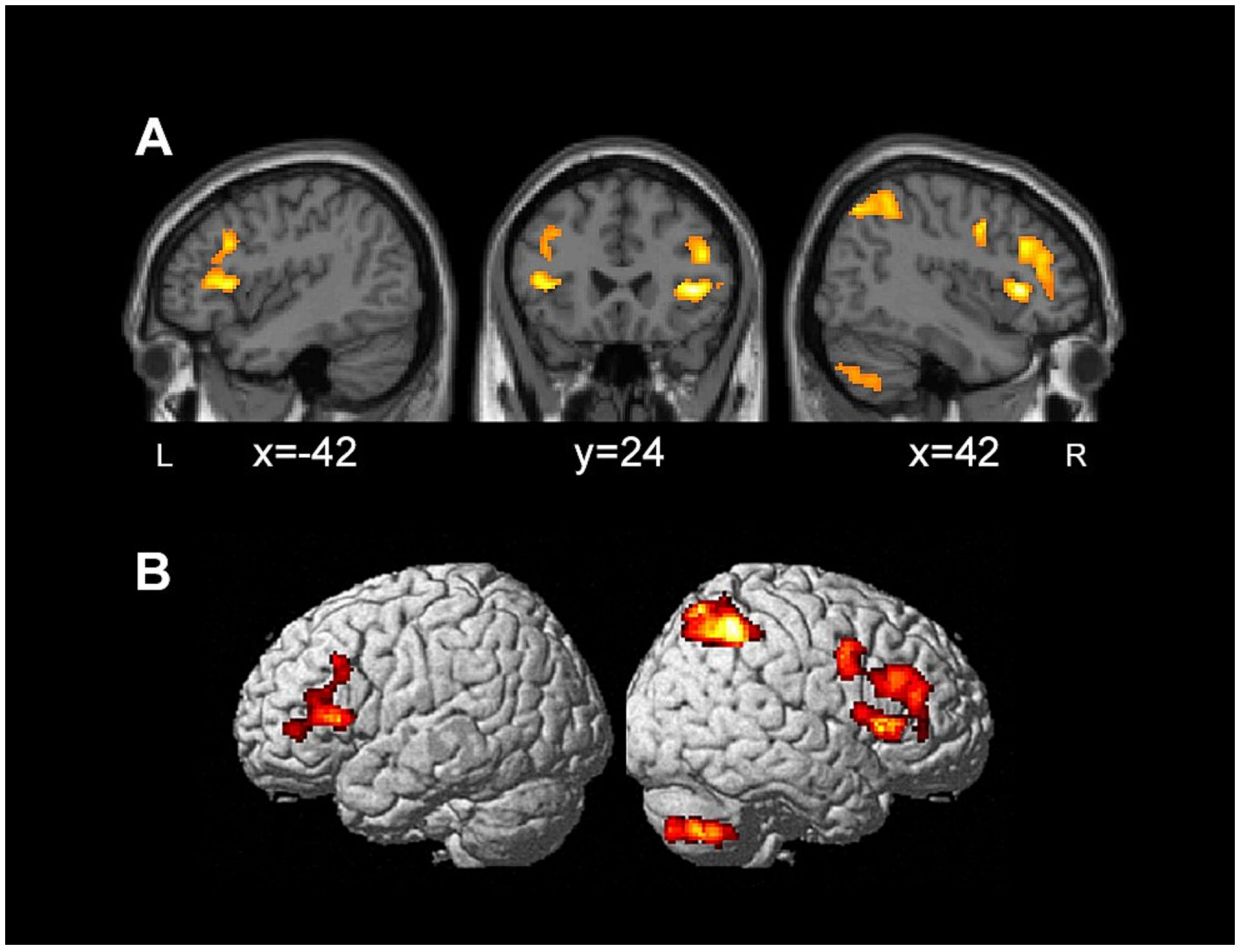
Brain regions with increased 18F-FDG PET glucose metabolism in patients with traumatic brain injury after rehabilitation, displayed on sagittal and coronal sections (A), as well as 3D renders (B).
